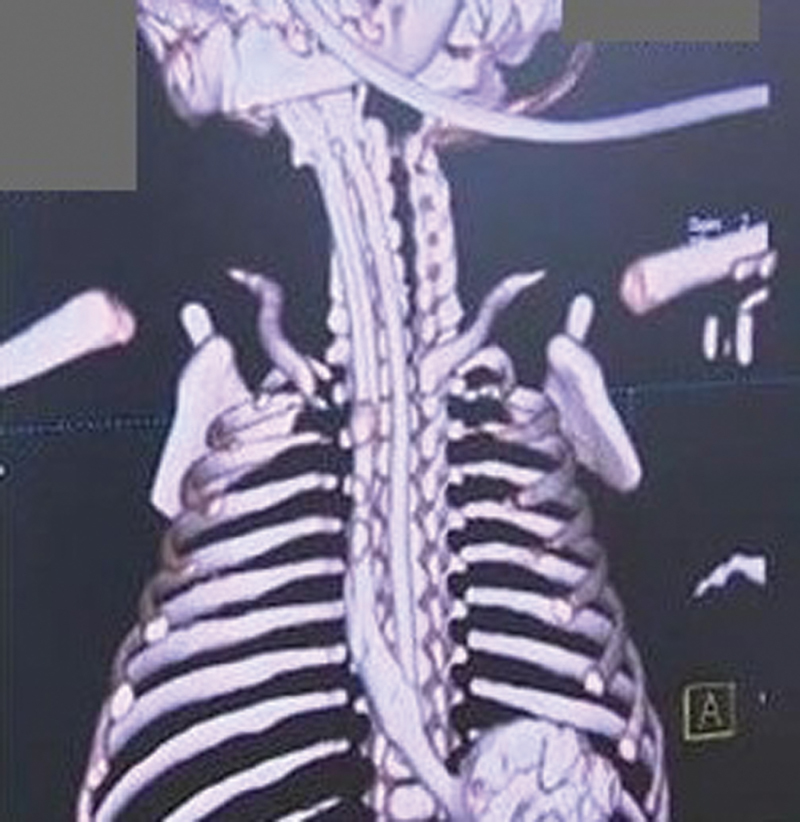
Computed tomography (CT) showing a second lumen along right the orthotopic esophagus.
